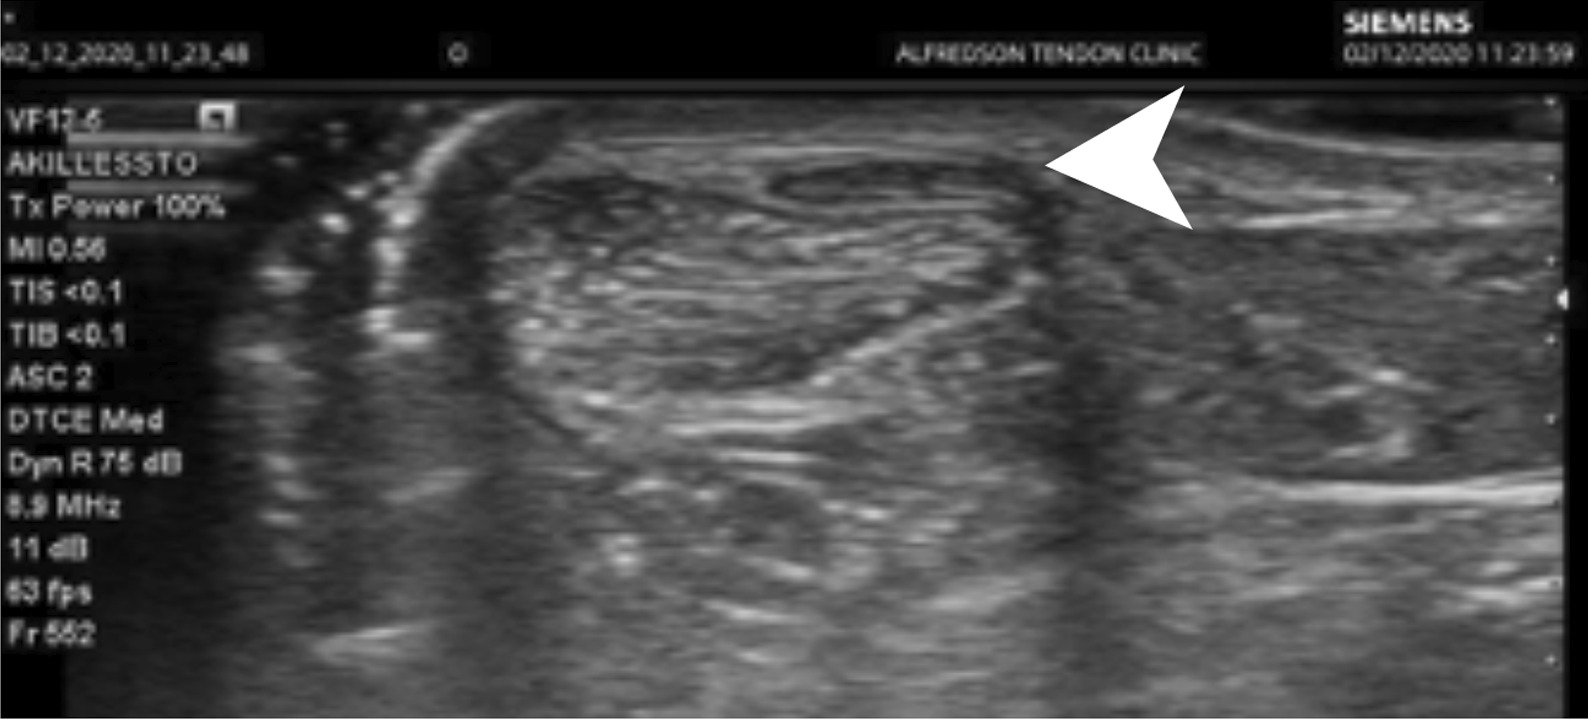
Grayscale ultrasound picture - transversal view showing a normal Achilles midportion and medial on the superficial side of the Achilles there is a plantaris tendon (arrow).
